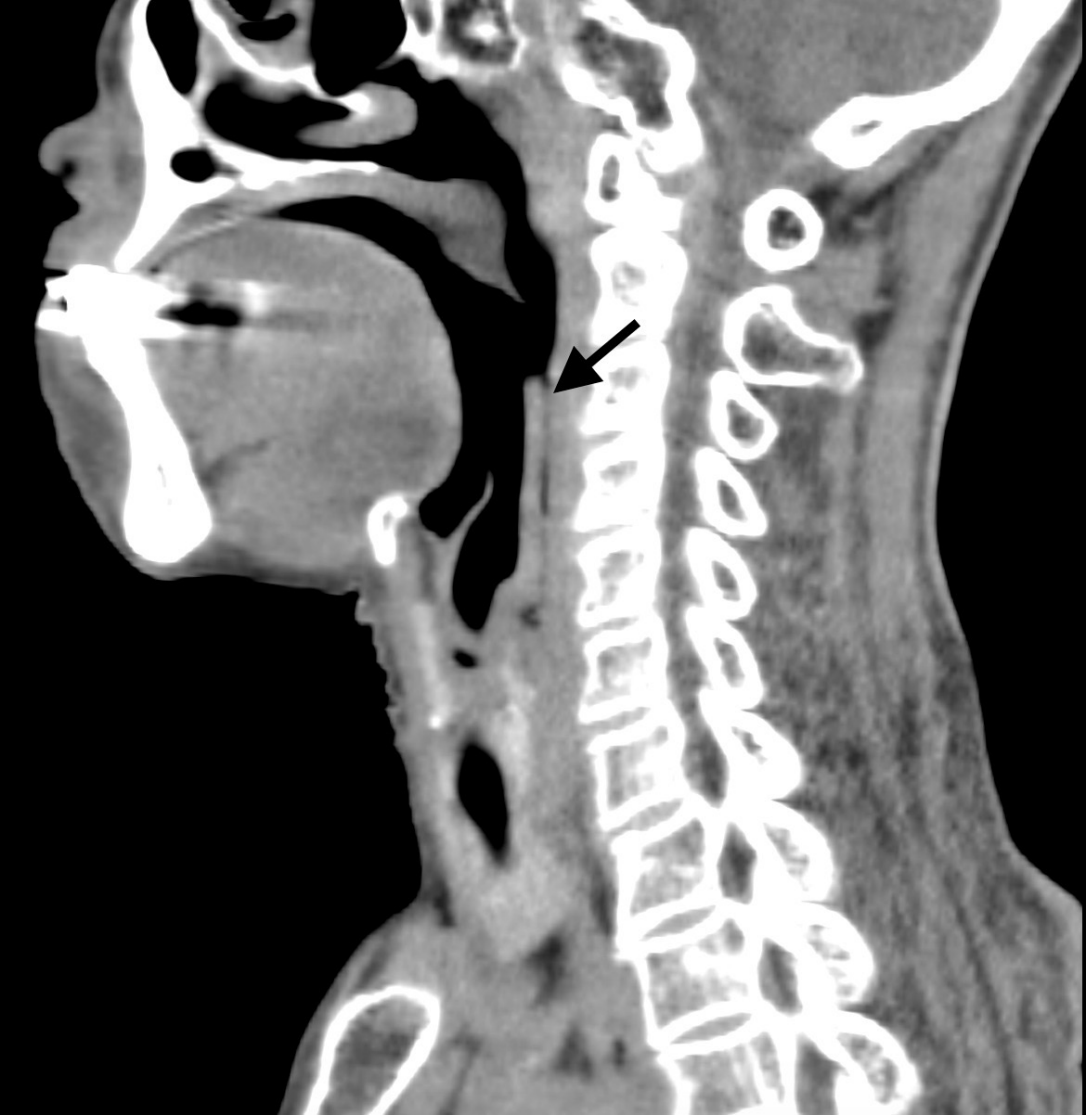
Sagittal view of computed tomography imaging showing the plate‐shaped object adhered to the posterior wall of the pharynx (arrow).
